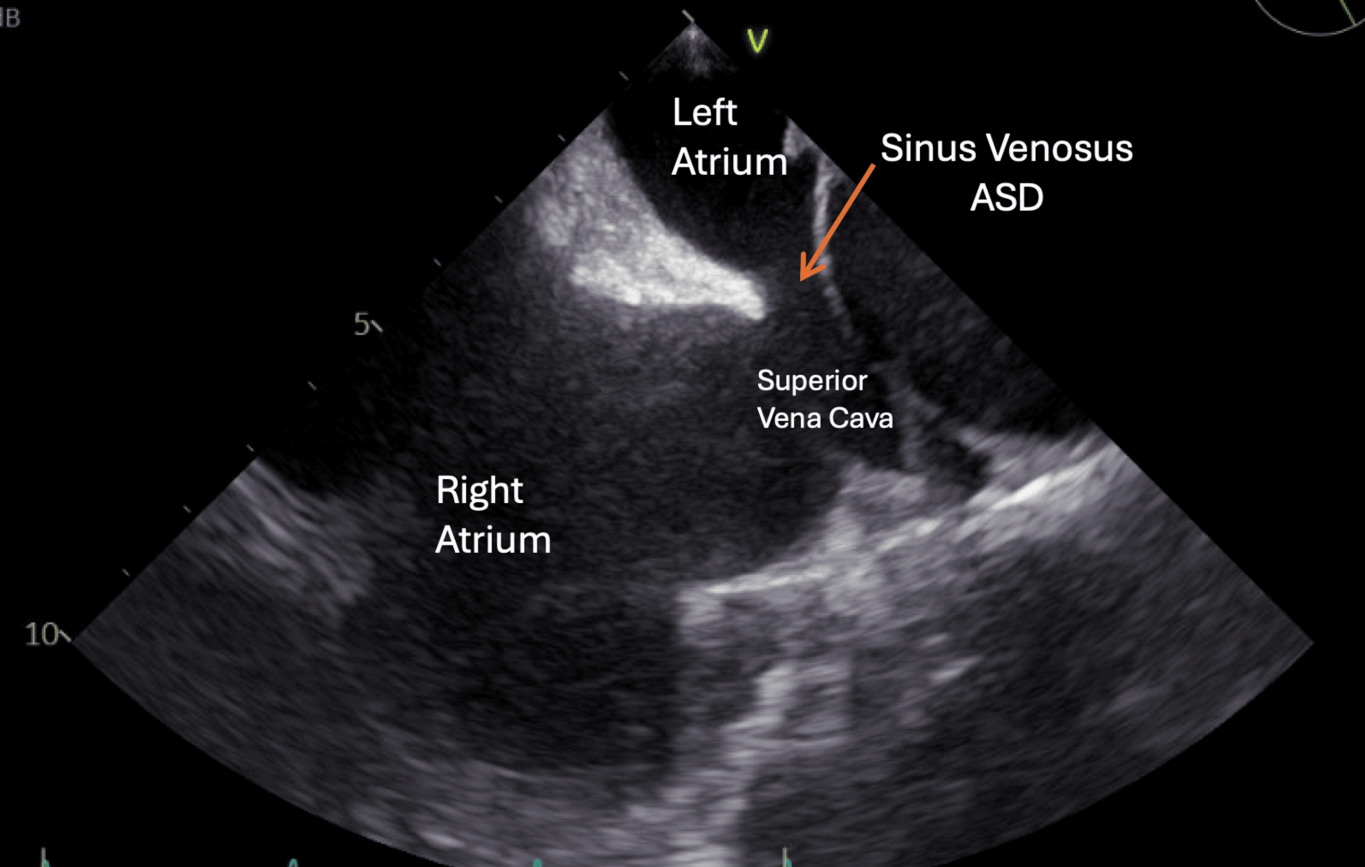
Transesophageal echocardiogram The bicaval view showed a sinus venosus ASD with associated PAPVR of right pulmonary veins to the SVC. ASD: Atrial septal defect; PAPVR: Partial anomalous pulmonary venous return; SVC: Superior vena cava
